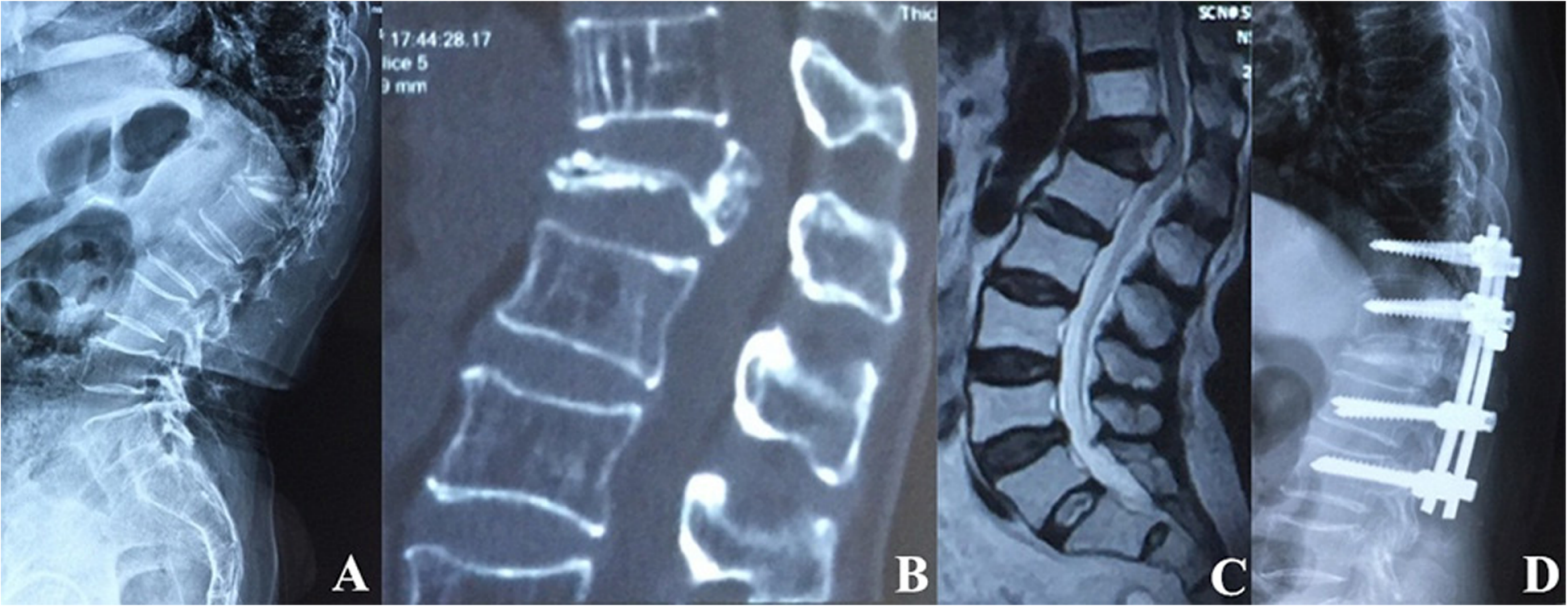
The patient was characterized by “dynamic instability and spinal stenosis and kyphosis deformity”. The surgical approach was posterior decompression and pedicle screw fixation and fusion. a–c The preoperative flexion lateral radiography, supine computed tomography reconstruction view, and magnetic resonance imaging, respectively. d Postoperative lateral radiography
